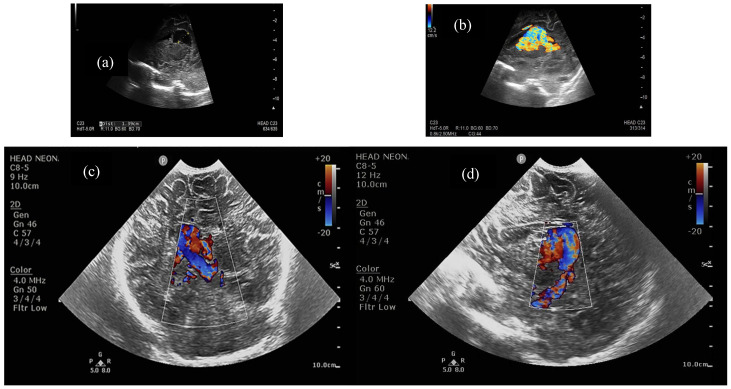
(a). Two-dimensional ultrasound close-up of the VoGM, (b). Color Doppler close-up of the VoGM, (c). Color Doppler in coronal transfontanellar section, (d). Color Doppler in parasagittal transfontanellar section. Median and right paramedian, supratentorial arteriovenous dilation of 10/28/20 mm, inferior to corpus callosum, with both arterial and venous supply, and suggestive appearance of an aneurysm of the Galen vein. Asymmetrical ventricular system, located on the midline. Focal lesions not visible at the level of the explorable cerebral parenchyma, except for small left parietal hematomas. Normally sized pericerebral spaces.
